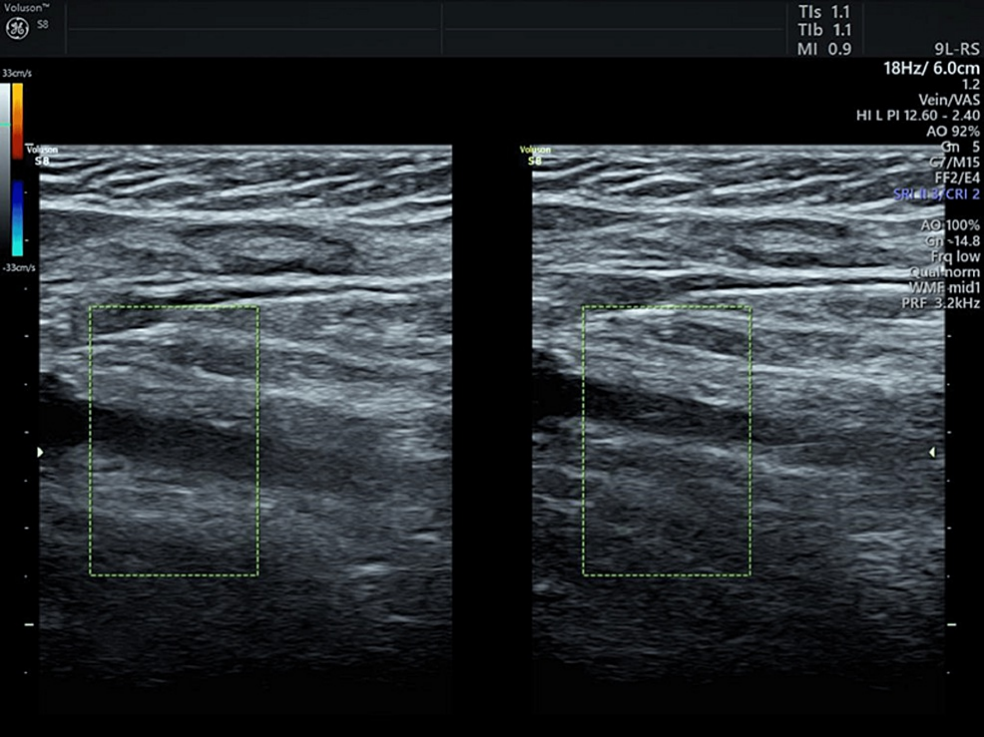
USG Doppler of the left lower limb showing a DVT in the superficial femoral vein, i.e., echogenic thrombus in distended vein and no color flow on Doppler USG: ultrasonography, DVT: deep venous thrombosis
